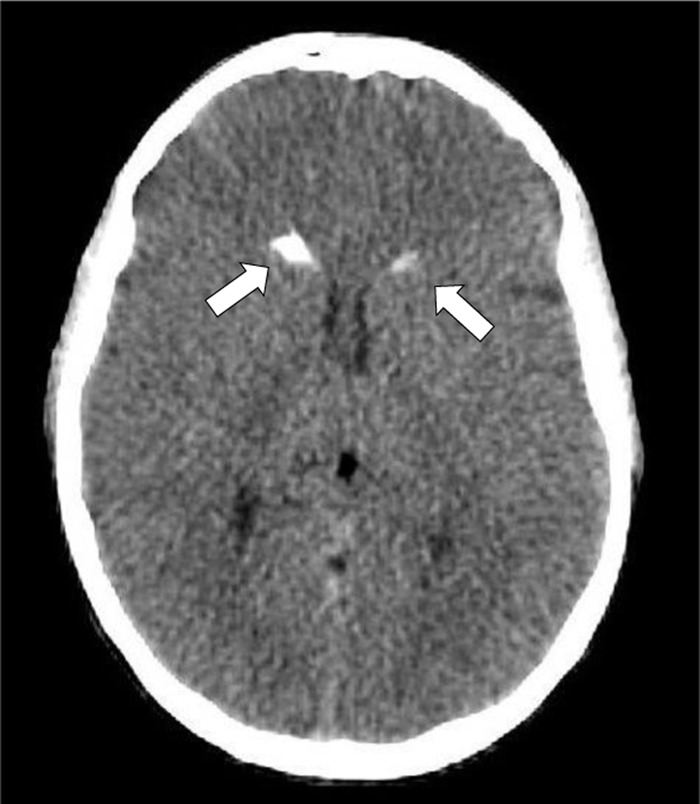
Brain window of low-dose head CT showing bilateral calcification in CN (white arrows). Figure adapted from a previous article [6].
